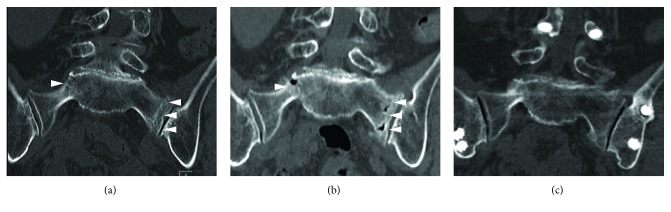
Coronal sacral CT images obtained at the first visit (a), admission (b), and final follow-up (c). The initial CT revealed nondisplaced bilateral fracture of the lateral mass of the sacrum (white arrow head). At the time of admission, CT showed slightly aggravated displacement of the fracture (white arrow head). CT at the final follow-up demonstrated bony union of the sacral lateral mass fracture. CT: computed tomography.
